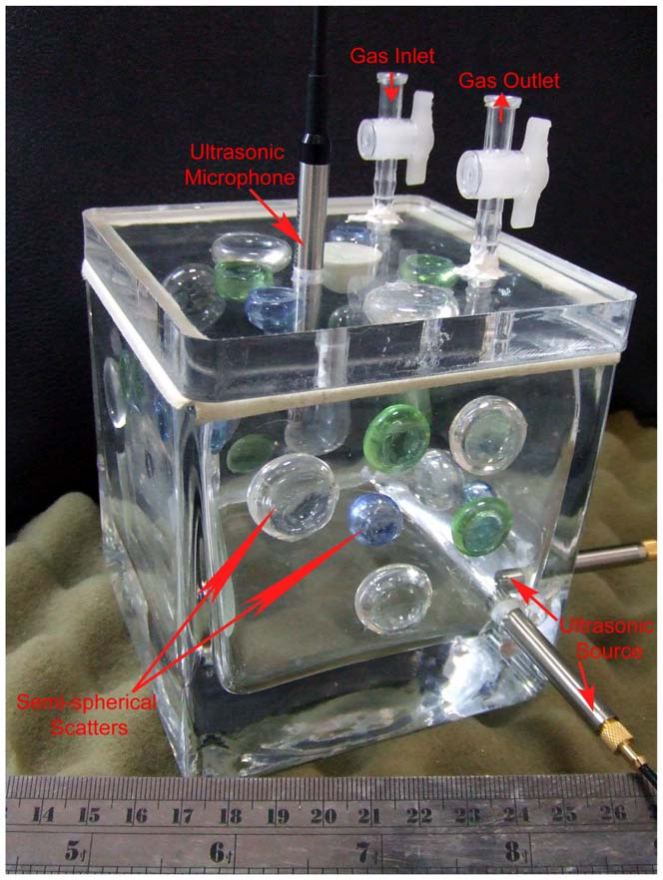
Reverberation chamber used for the ultrasonic absorption measurements. The ultrasonic source is deployed in a chamber corner, and the ultrasonic microphone can be moved from the lid up-and-down to warrant spatial averages. Many semi-spherical scatters are also deployed on the interior surfaces to achieve a diffuse sound field within the frequency range of interest. Medium exchange by nitrogen is carried out by one of the gas inlet/outlets.
